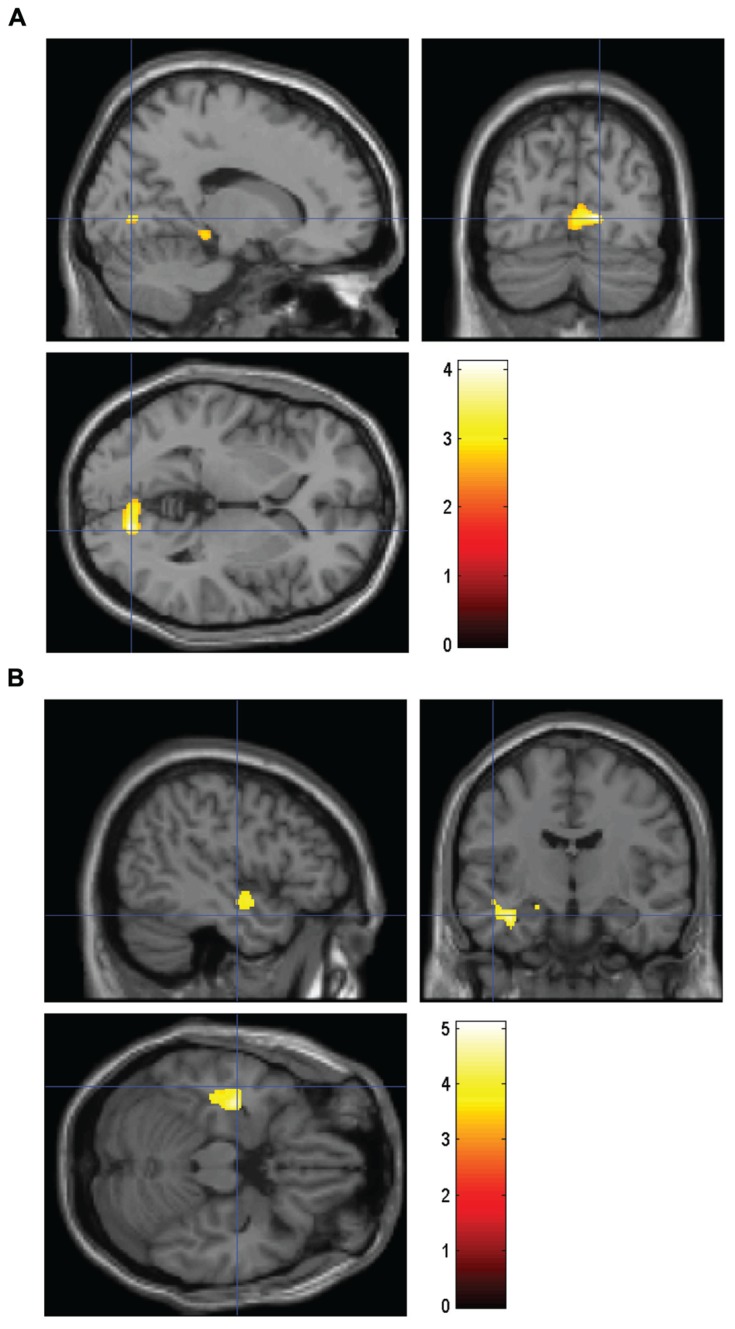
Volume comparing HS/LE participants to HE/LS participants. (A) Regions of relatively greater volume in HS/LE compared to HE/LS projected onto a T1-weighted image for the right parahippocampus (14 -75 1), the right hippocampus (11 -4 -15), the right cuneus (8 -79 18), and the left orbitofrontal cortex (-15 9 -20). (B) Regions of relatively decreased volume in HS/LE compared to HE/LS projected onto a T1-weighted image for the left inferior temporal cortex (-41 -16 -17), the left insula (-48 -4 -11), and the left amygdala (-18 -15 -14). The color bar represents the *t*-score.
